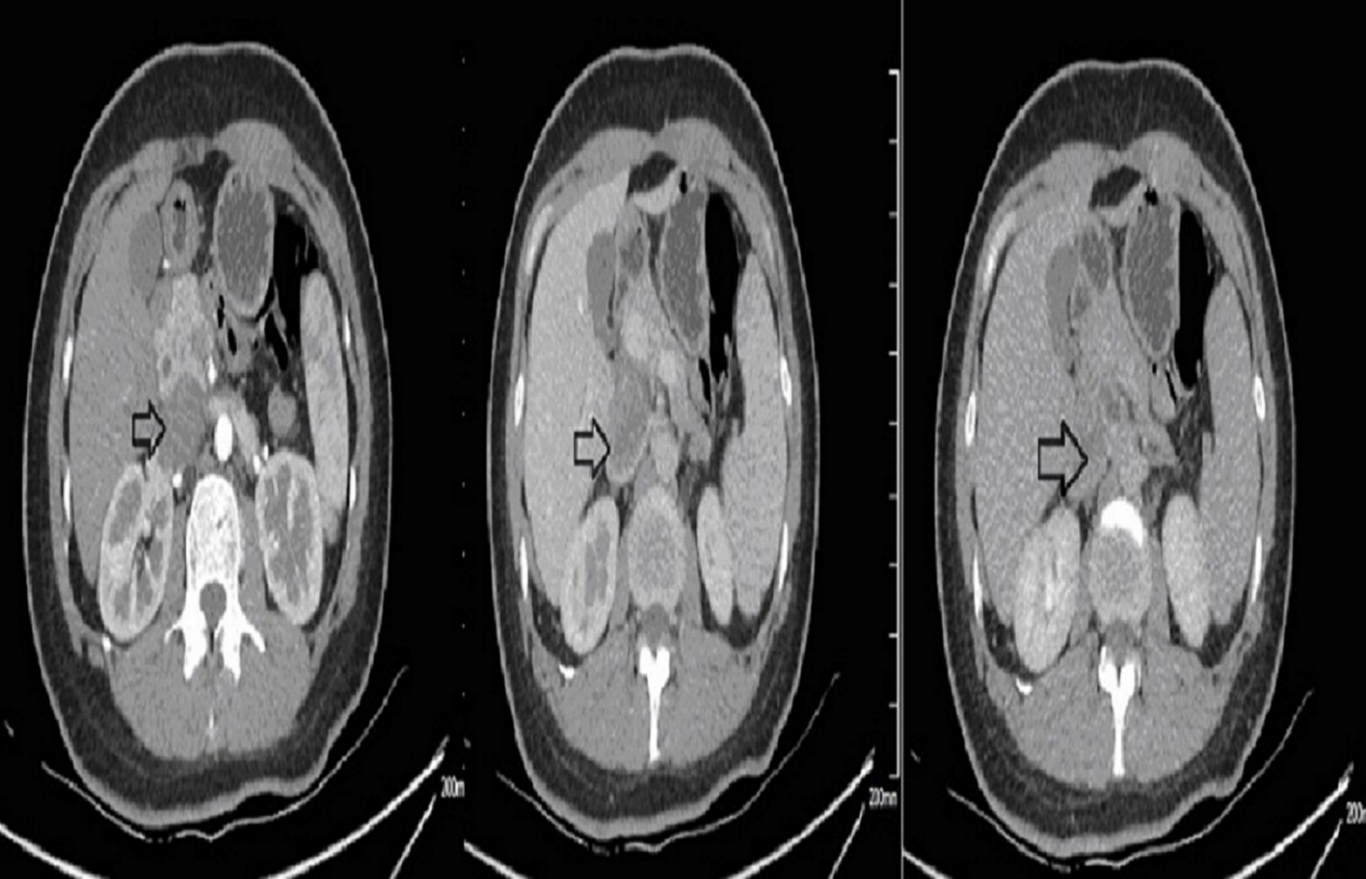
Preoperative contrast-enhanced CT images. The border between the tumor and IVC was indistinct.
